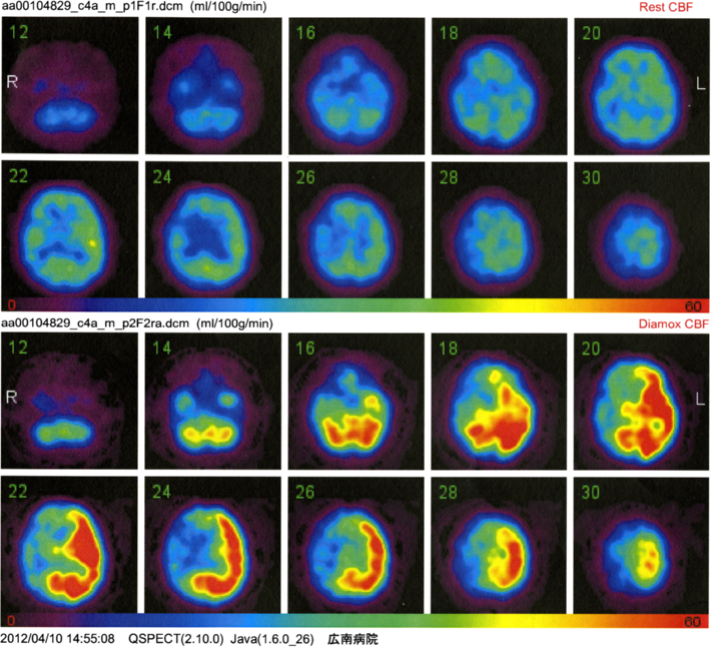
Iodine-123 N-isopropyl-p-iodoamphetamine single photon emission computed tomography scans revealing slight reduction of cerebral blood flow in the right hemisphere, and the vascular reserve capacity was markedly reduced after injection of acetazolamide.
